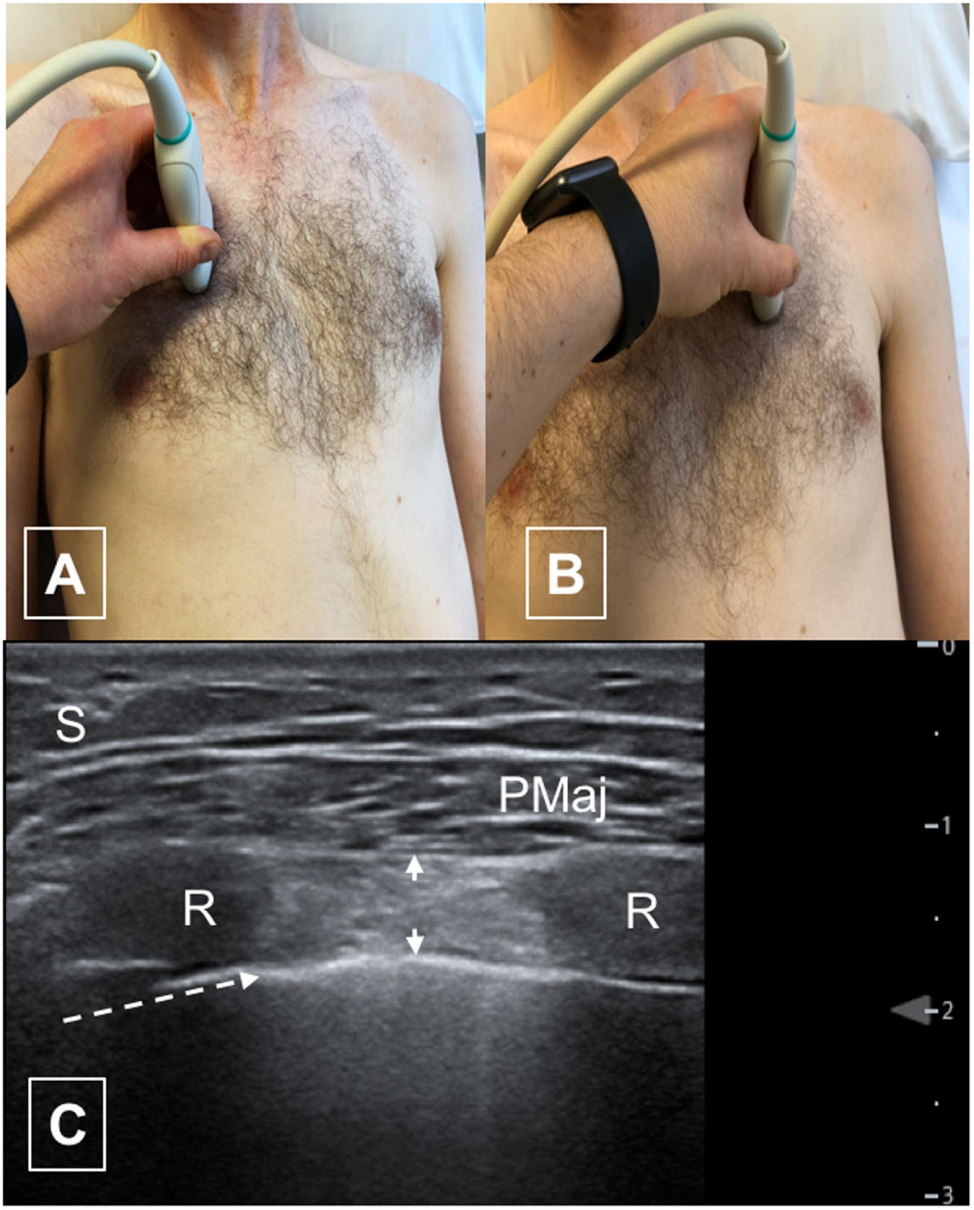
Parasternal intercostal ultrasound. (A) Position of probe for right second intercostal space. (B) Position of probe for left second intercostal space. (C) Typical ultrasound image of parasternal intercostal muscle (left 2^nd^ intercostal space. S – subcutaneous fat PM – pectoralis major muscle. R – rib Arrow heads – outline of intercostal muscle. Dotted arrow – pleural line.
